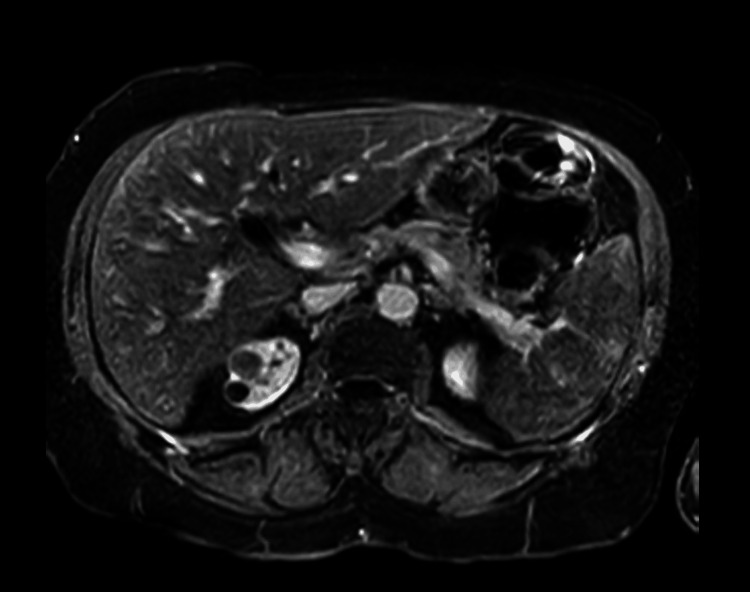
MRI of the abdomen for further visualization of the pancreas to rule out insulinoma
